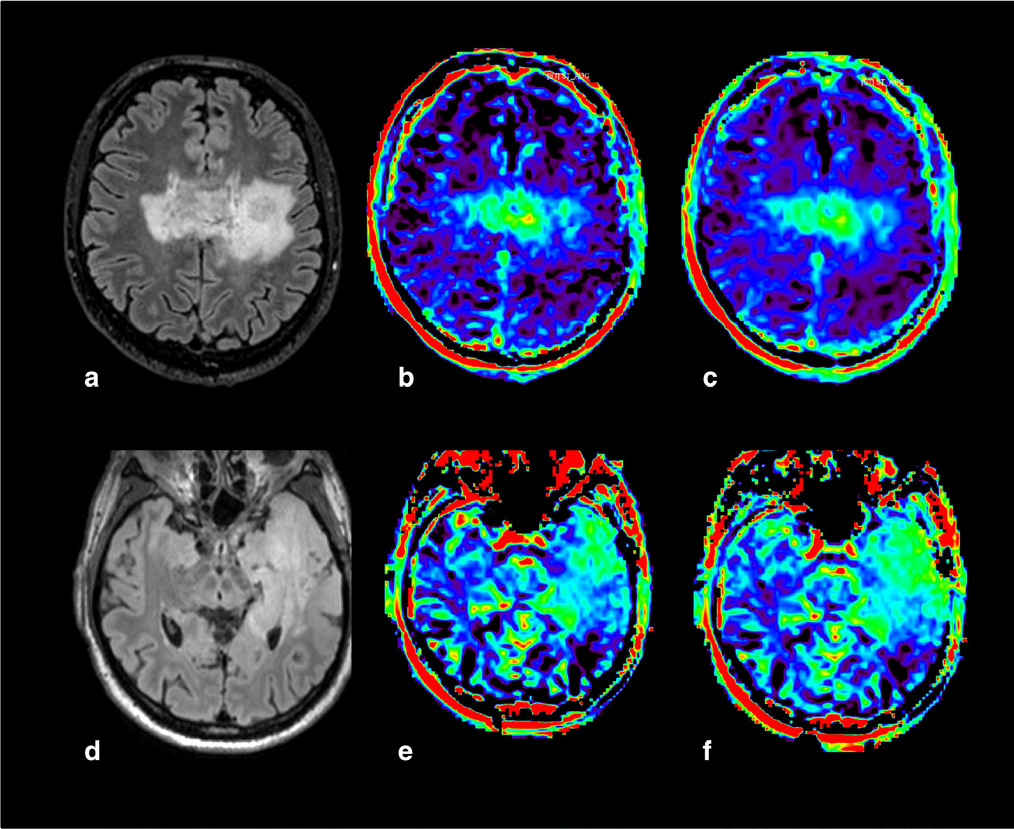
Example of motion artifacts in two glioblastomas (IDH-wildtype, grade 4) (*case courtesy of Sotirios Bisdas and Laura Mancini, University College London*). (a) 3D FLAIR of the case depicted on Figure 7. (b) APTw map before motion artifacts correction. (c) APTw map after an adequate motion artifact correction. (d) 3D FLAIR. (e) APTw map before motion artifacts correction. (f) APTw map after an inadequate motion artifact correction due to too strong kinetic movements. 3D, three-dimensional; ADC, apparent diffusion coefficient; APTw, amide proton transfer-weighted; FLAIR, fluid attenuated inversion recovery.
